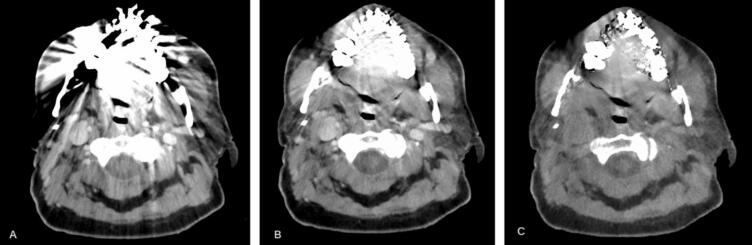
Patient 9 PCD-CT reconstructions. A) Virtual monoenergetic image of 70 keV (VMI70) without iterative metal artifact reduction (iMAR); B) VMI70 with iMAR; C) Relative electron density (RED) image with iMAR. All images shown in the abdomen window (CT values between −125 and 225 HU). (For interpretation of the references to colour in this figure legend, the reader is referred to the web version of this article.)
